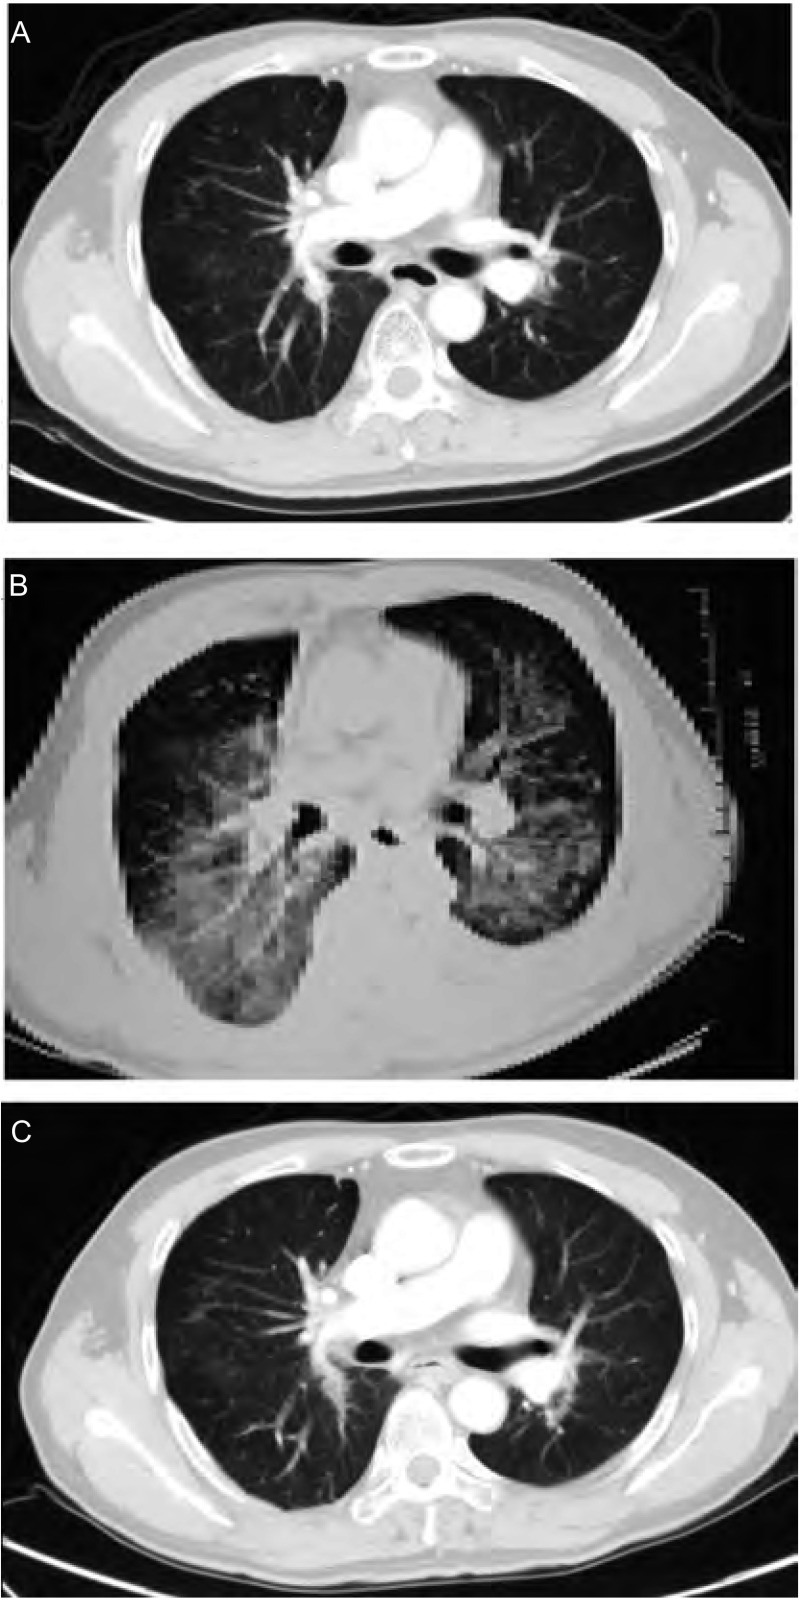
病例1厄洛替尼治疗前后及糖皮质激素治疗后胸部CT变化 Chest CT scans before and after erlotinib treatment and after corticosteroid treatment. A: Chest CT scan before erlotinib treatment; B: Chest CT scan after 24 days of erlotinib treatment; C: Chest CT scan after 7 days of corticosteroid treatment.
